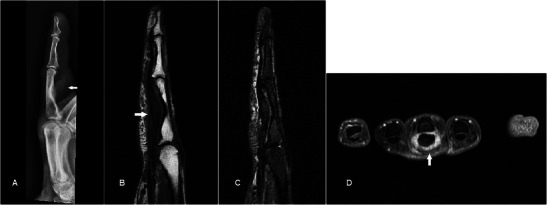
Chronic calcific tendinopathy in a patient presenting with 10-year history of focal swelling along the volar aspect of the left middle finger.(a) Plain radiograph shows well-defined scalloping along the volar aspect of the middle finger proximal phalanx with an overlying striated pattern of calcification (arrow). (b) T1w sequence shows focal swelling of the flexor digitorum longus tendon (arrow) causing scalloping of the underlying bone. No discrete mass is seen. (c) There is a mild increase in signal in the tendon on T2-FS sequence suggesting tendinosis. There is bony scalloping but no significant marrow oedema, suggesting a chronic process. (d) Diffuse thickening and intense enhancement of the tendon sheath is seen, reflecting an element of active tenosynovitis (arrow)
